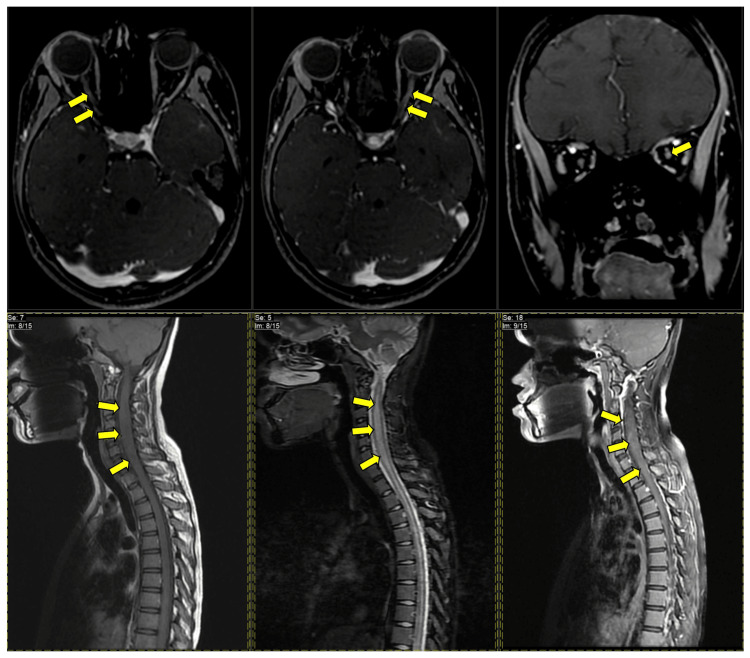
Gadolinium-contrasted T1-weighted MRI orbits (Top 3 slices-axial & coronal views) & T1-weighted, T2-flair, and gadolinium-contrasted T2 MRI spine images (in sequence) (Bottom 3 slices-sagittal view)
